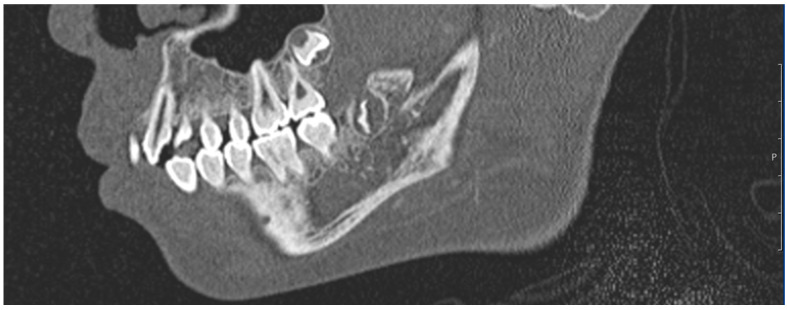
CT image of a 14-year-old subject with wisdom teeth 18 and 48.
